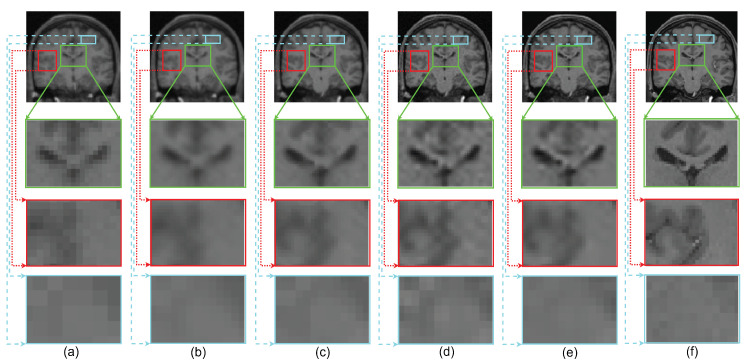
Demonstration of reconstruction quality of different kinds of regions/patches, region with edges (green boxes), region with texture (red boxes), and smooth region (cyan boxes), after super resolving real MR images degraded with downsampling factor 2 and 2% noise, using different algorithms. (a) NN interpolation of noisy LR image, (b) spline interpolation of denoised LR image, (c) NLM3D [13] applied on denoised LR image, (d) LRTV [12] applied on denoised LR images, (e) the proposed work applied on a noisy LR image, and (f) the original noise-free HR image.
